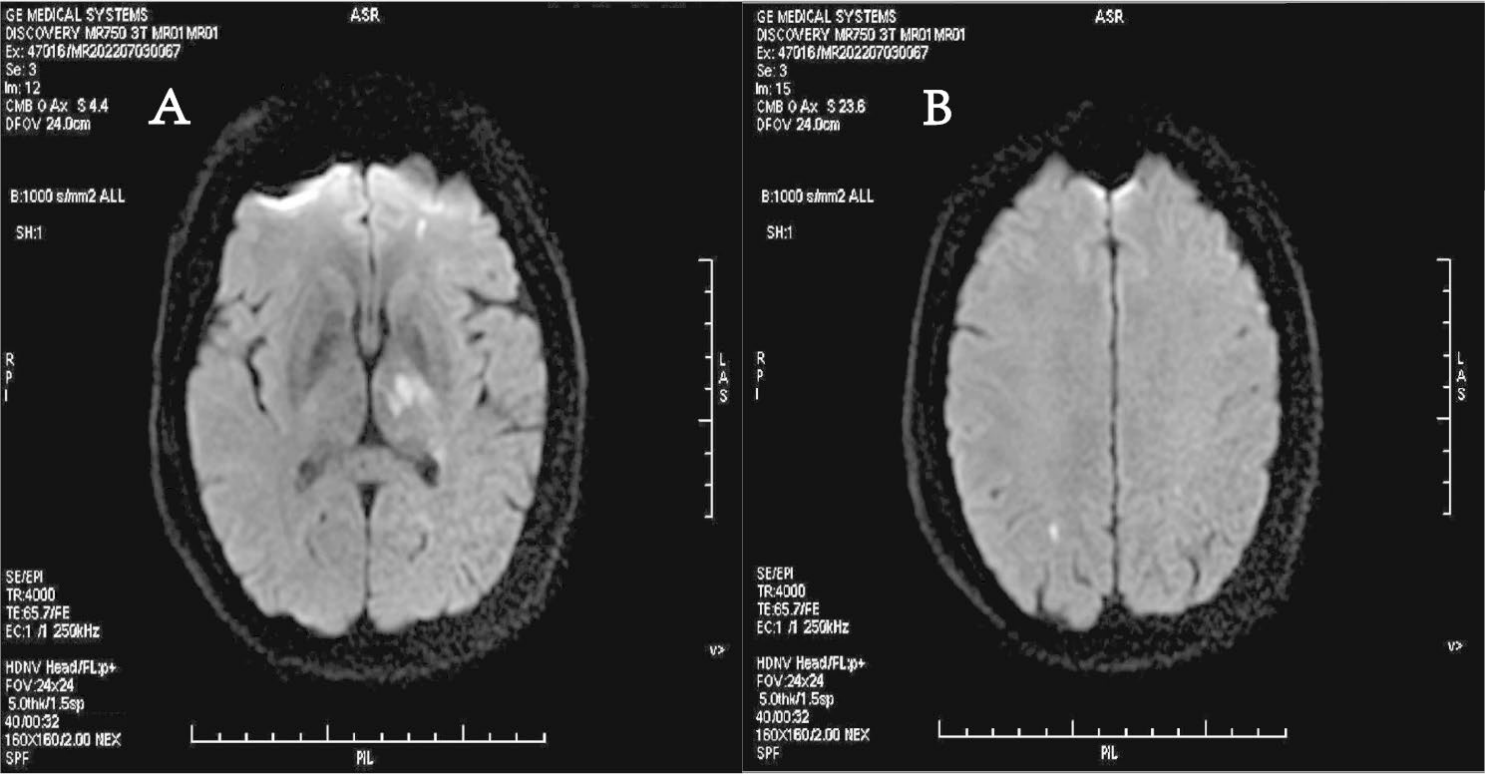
Brain magnetic resonance imaging (MRI) showed patchy and punctate slightly high signals in left basal ganglia and right parietal lobe
